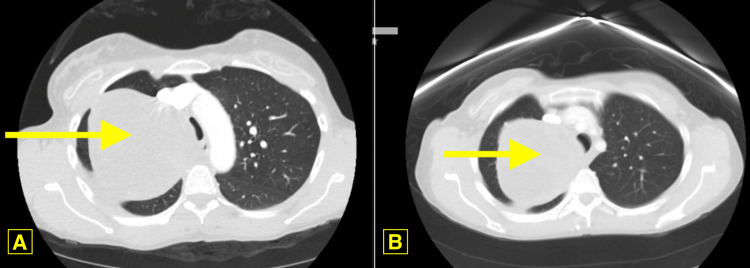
A computed tomography angiography of the chest (A), revealed a large, heterogeneous solid mass in the right apical region, measuring approximately 10.6 cm × 11.3 cm × 11.8 cm, that was contiguous with the pleural surface. The lung mass was found to have grown slightly since the previous CT chest, taken four years prior, which had measured to be 10.4 cm × 8.5 cm × 10.5 cm, as shown in the right panel (B).
